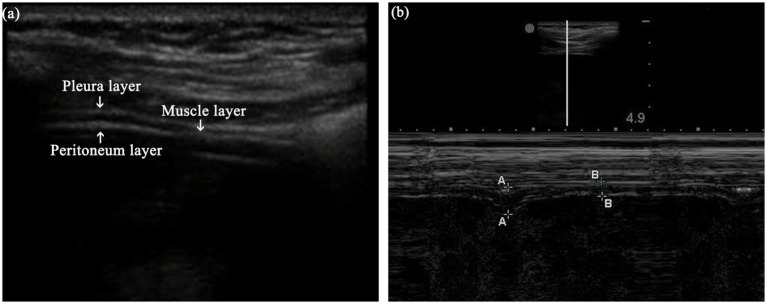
Measurement of diaphragm thickness, (a): B ultrasound manifestation of diaphragm: “three-line” structure indicated by the arrows; (b) the respiratory diaphragm thickness change curves in the M-mode, AA: end-inspiratory diaphragm thickness (Tdi^ins^), BB: end-expiratory diaphragm thickness (Tdi^exp^).
